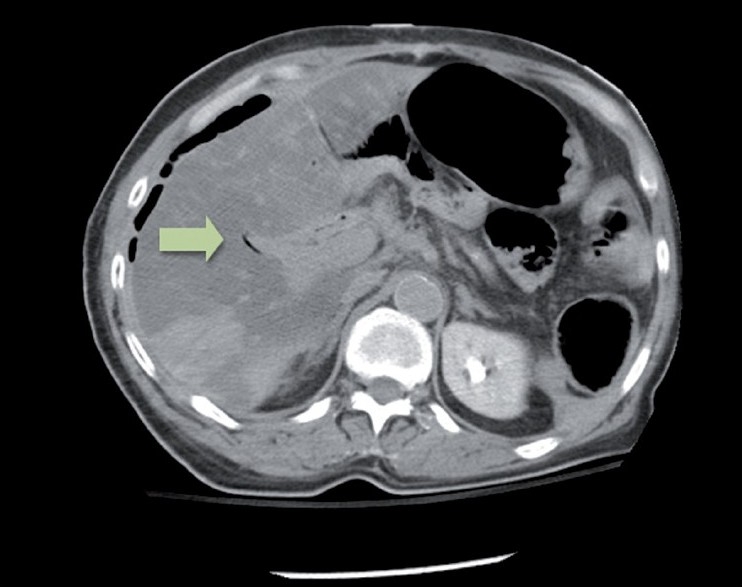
CT scan of the abdomen prior to the patient’s current admission demonstrating pneumobilia but no intraperitoneal free air
